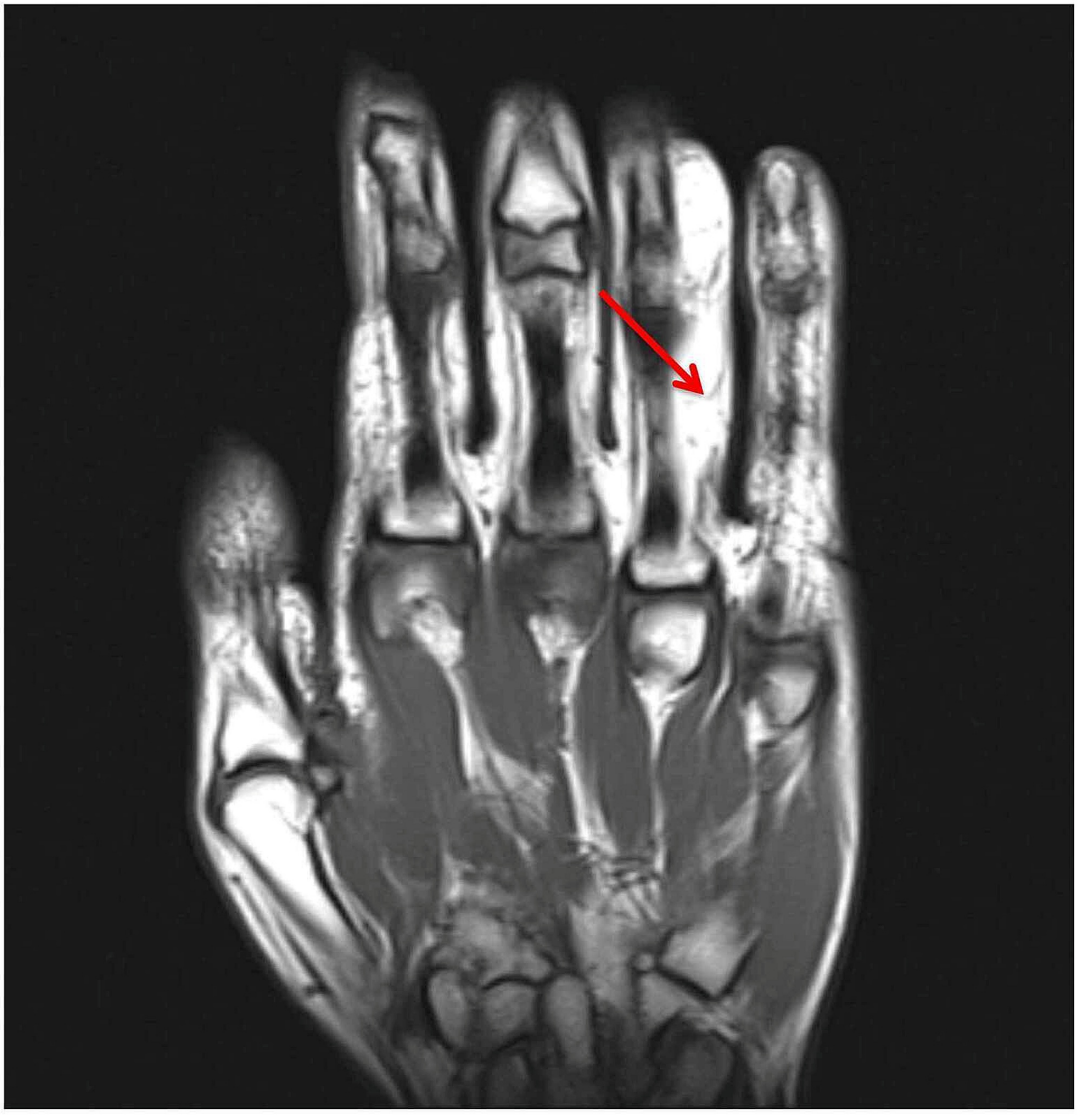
MRI image of the right hand without gadolinium contrast enhancement. The mass demonstrated a hyperintense signal on coronal T1-weighted imaging (arrow) MRI: magnetic resonance imaging
